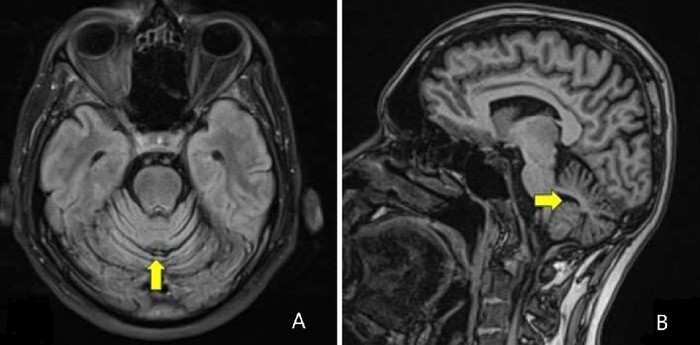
(A) Axial magnetic resonance imaging (MRI) of the brain shows gross cerebellar atrophy (arrow). (B) Sagittal MRI of the brain shows dorsal and anterior vermian and paravermian cerebellar atrophy (arrow).
